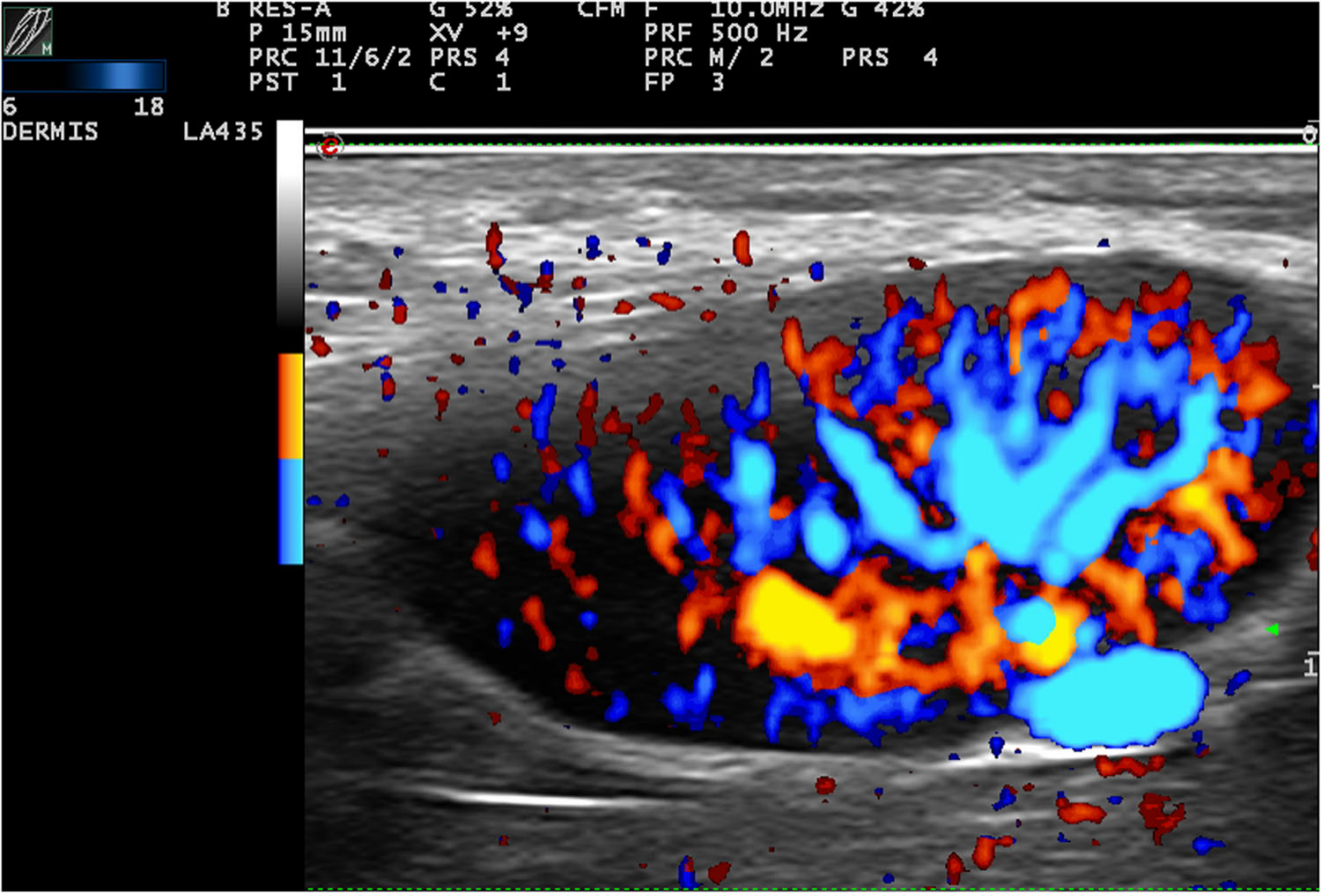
Case 3. High‐frequency ultrasound pattern of inflammation in an enlarged lymph node.
